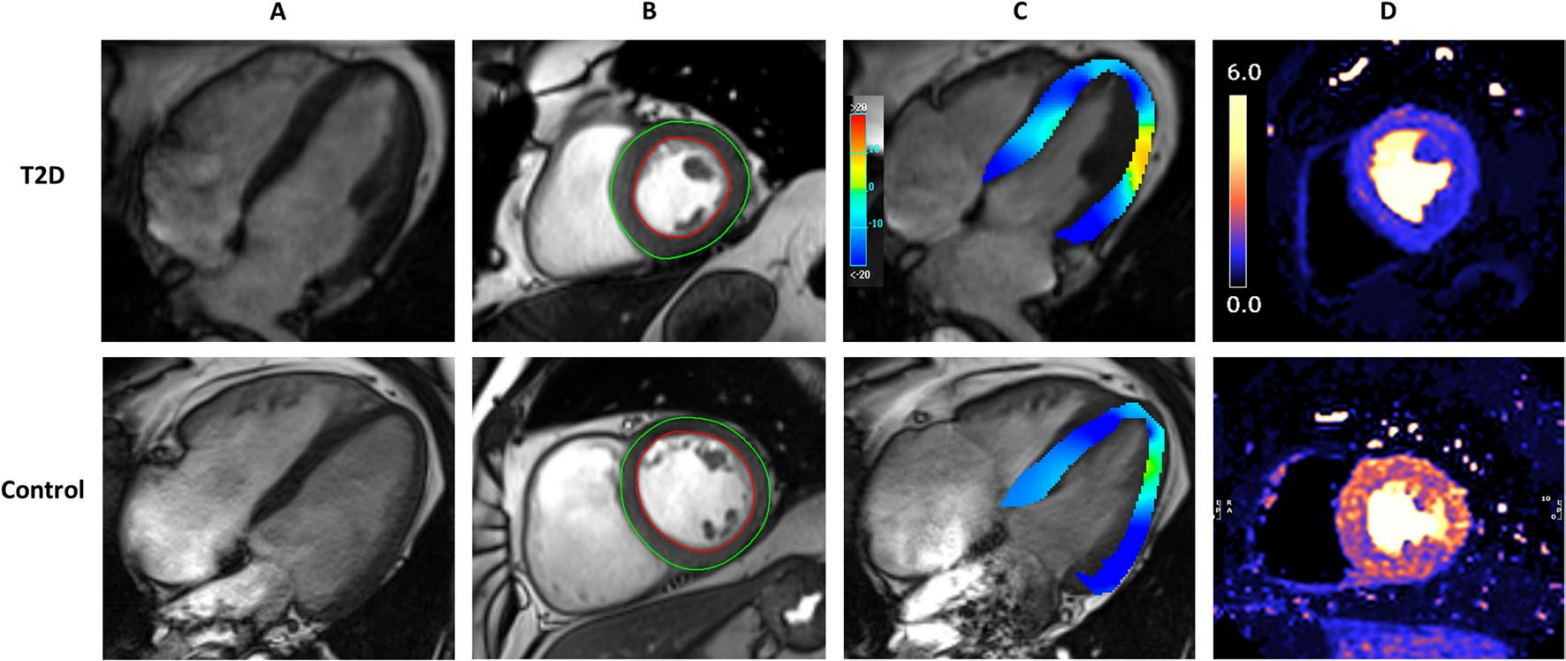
MRI images displaying the 4-chamber view (A) and mid- ventricular short-axis slice (B) during diastole, global longitudinal strain assessment (GLS) (C), and stress myocardial perfusion map in mid-ventricular short-axis slice (D). Top row images were from a 59-year-old male with type 2 diabetes (T2D), BMI of 40 kg/m^2^; left ventricular mass (LVM) 146 g, left ventricular end-diastolic volume (LVEDV) 122 mL, left ventricular mass to volume ratio (LVM/V) 1.2 g/mL, GLS 13.0%, stress myocardial blood flow (MBF) 1.2 mL/min/g, rest MBF 0.6 mL/min/g, and myocardial perfusion reserve (MPR) 2.0. The bottom row images were from a 64-year-old male non-diabetic control, BMI of 32 kg/m^2^; LVM 179 g, LVEDV 223 mL, LVM/V 0.8 g/mL, GLS 15.3%, stress MBF 2.2 mL/min/g, rest MBF 0.6 mL/min/g, and MPR 3.7. Note for GLS with darker blue indicates greater shortening and higher strain
